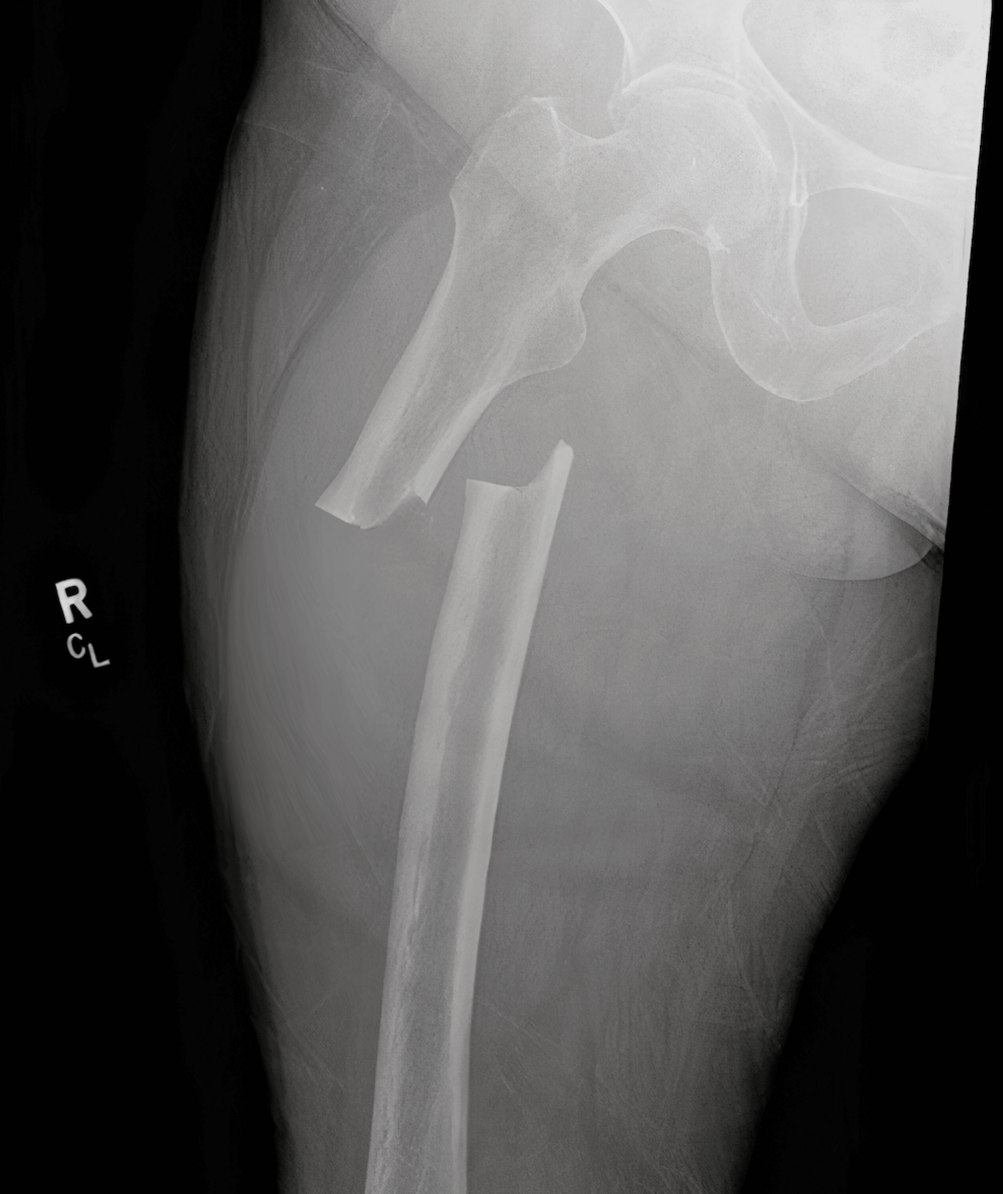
Preoperative radiograph showing a transverse subtrochanteric femoral fracture with medial cortical spike and lateral cortical thickening. R: right, CL: contralateral.
